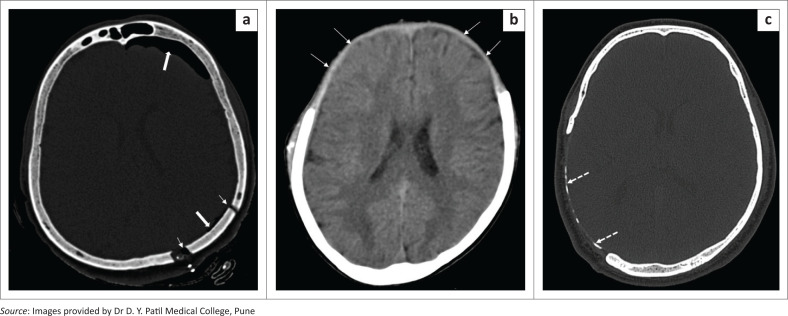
Axial bone window CT brain image (a) demonstrates a left parietal craniotomy (short white arrows) in a patient who underwent surgery for a left parietal space occupying lesion. Note the post-operative pneumocephalus in the left frontal and parietal regions (thick white arrows). Axial soft tissue window CT brain image (b) reveals bifrontal craniectomy with a normal meningogaleal complex seen as a slightly hyperdense linear structure separating the subcutaneous layer and the subarachnoid space (white arrows). Axial bone window CT image in another patient (c) indicating a right parietal craniectomy with chronic calcifications of the meningogaleal complex (dashed arrows).
